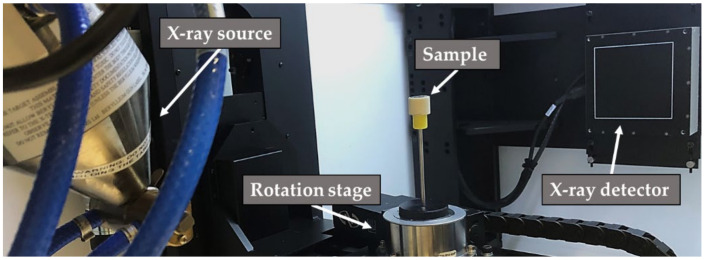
Setup of CT scanning with sample.
